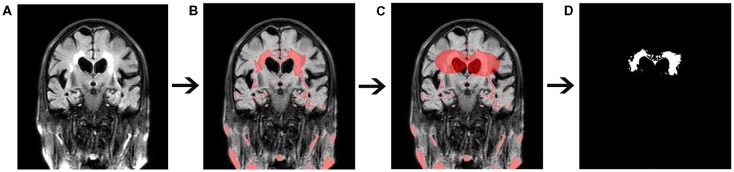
Quantitative measurement of white matter hyperintensity (WMH) volume. (A) Original fluid-attenuated inversion recovery (FLAIR) image. (B) The high-signal area. (C) A sketch of the affected WMH area. (D) Extraction of the affected WMH area.
